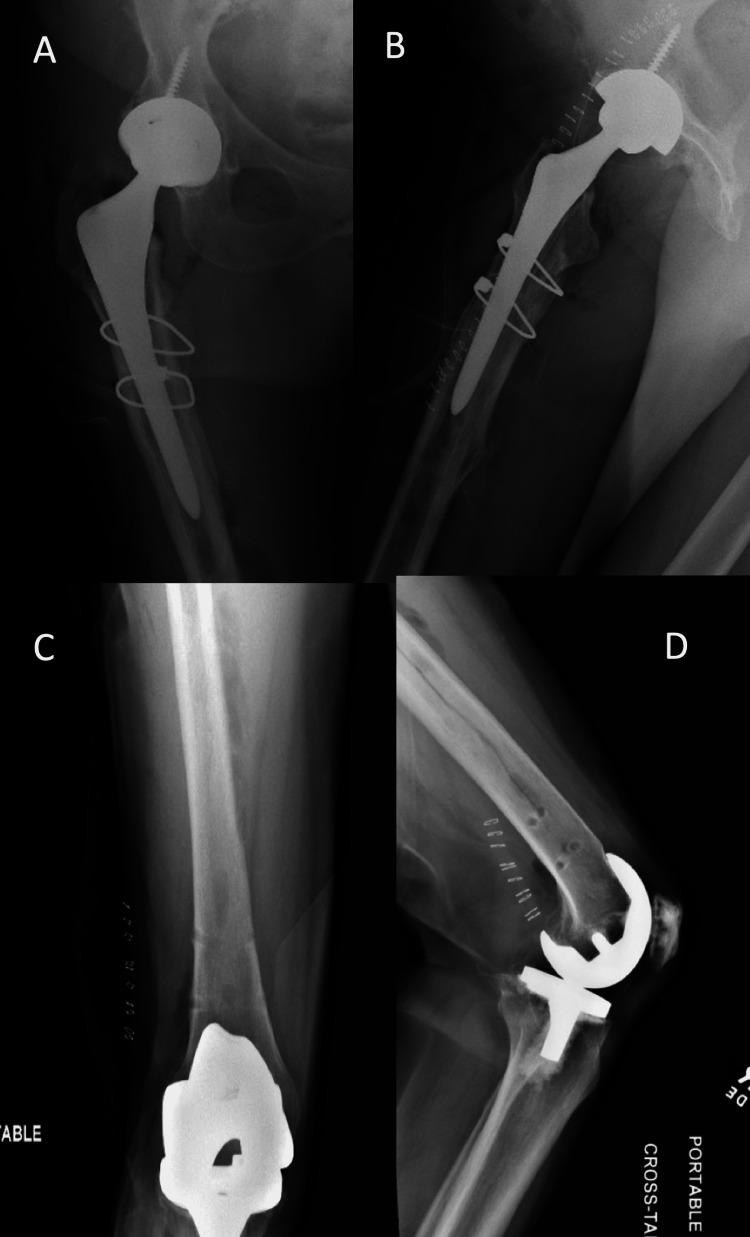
Immediate postoperative radiographs Anteroposterior (A,C) and lateral (B,D) immediate postoperative radiographs of the right hip and femur. There is a total hip arthroplasty secured with two cables. The distal interlocking screws have been removed, and there is a linear lucency seen advancing superiorly from the more proximal screw hole along the medial femoral shaft.
